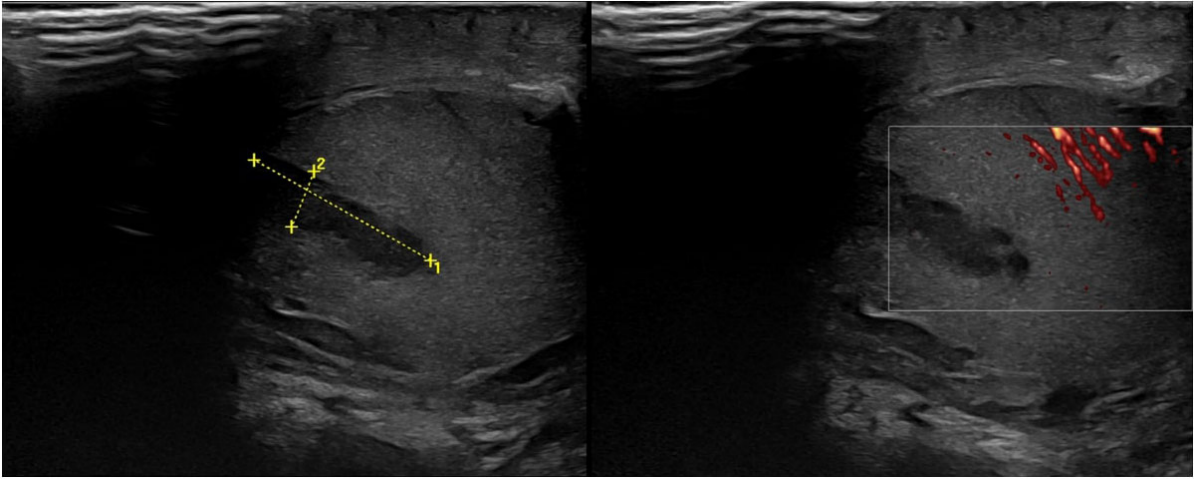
Scrotal ultrasound showing a 1.7/0.5 cm mass in the upper pole of the right testicle
